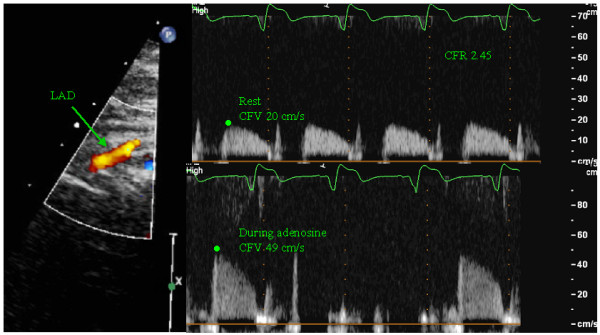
Coronary flow reserve measurement. To the left a color Doppler image of the mid-distal part of the LAD. The top image shows the PW recording of the blood flow velocity in the LAD during rest and the bottom shows blood flow during adenosine infusion. The scale has been changed from rest to hyperemia. The marking represents peak diastolic flow velocity. Here the CFR is 2.45.
